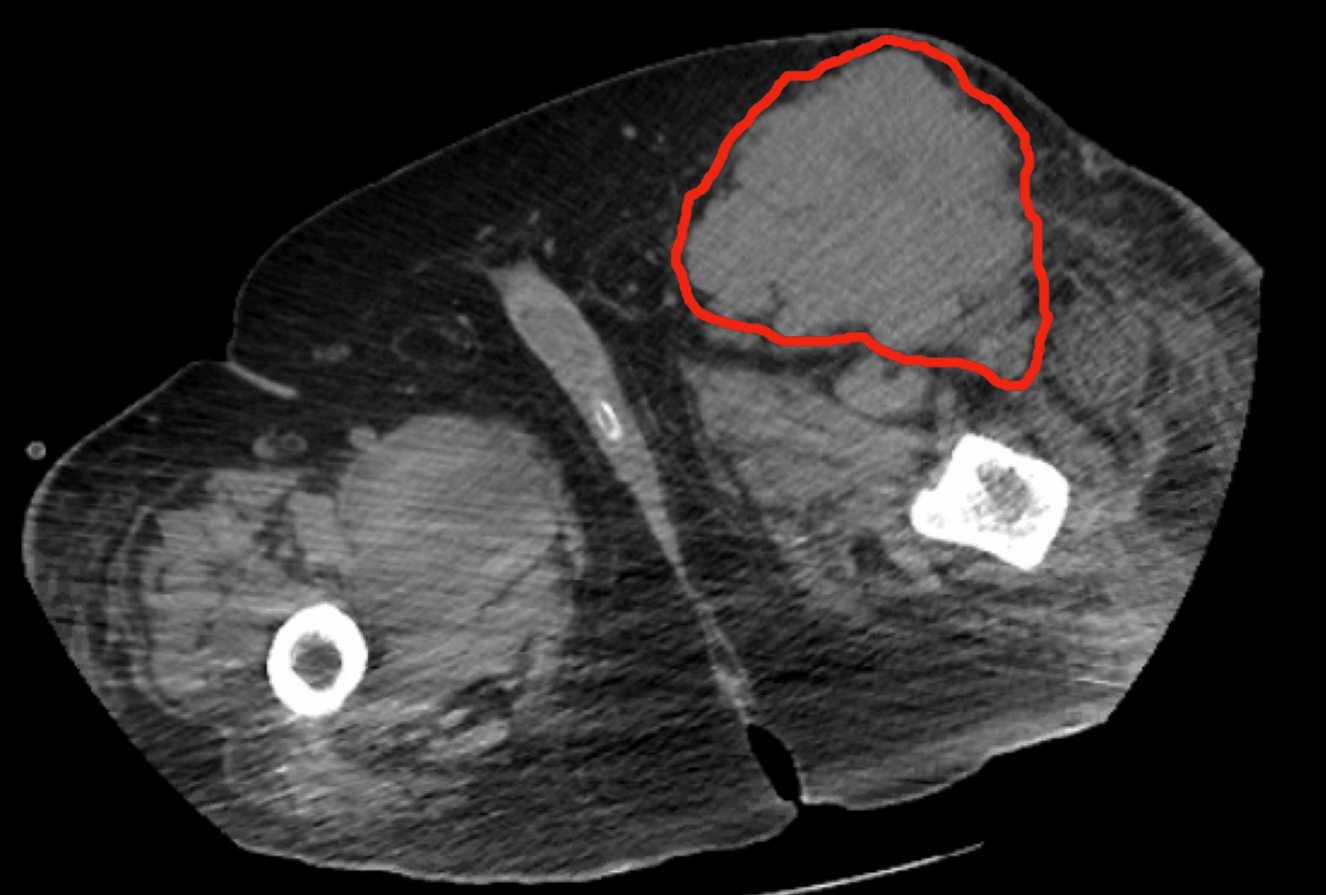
Pelvic CT showing left external iliac and inguinal chain lymph node mass (outlined in red). Pelvic computed tomography (CT) scan showing a lymph node mass involving the left external iliac and ipsilateral inguinal chains (outlined in red), with a maximum diameter of 11 cm. The largest inguinal lymph node demonstrates partial necrosis, raising high suspicion for malignancy.
